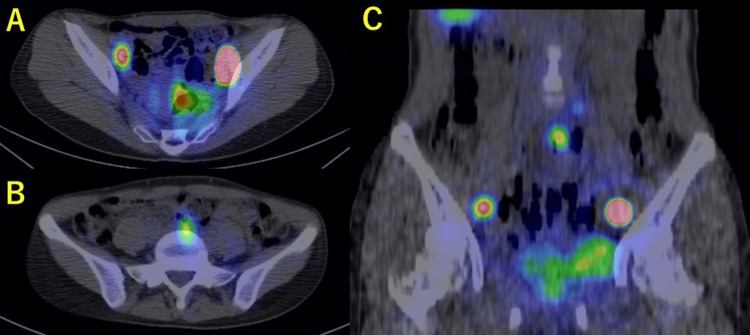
Sentinel lymph node identified by SPECT/CT. (A and C) Bilateral obturator lymph node; (B and C) presacral lymph node. SPECT/CT, single-photon emission computed tomography combined with computed tomography
